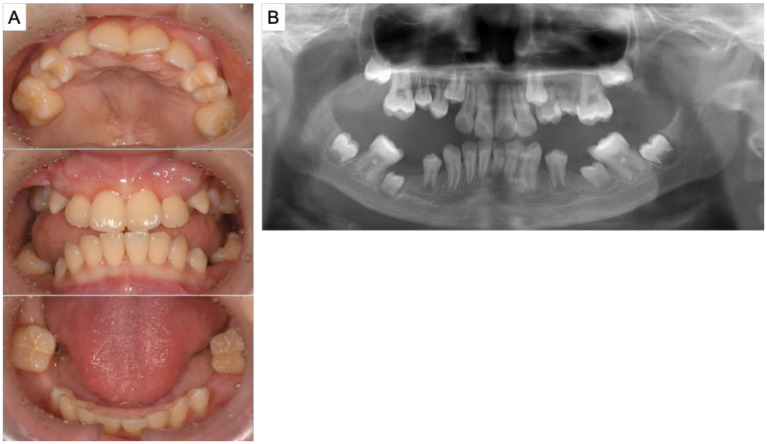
Images at the age of 10 years, showing improvement in the inflammation of the periodontal tissues. (A) Intraoral photographs. (B) Panoramic radiograph.
